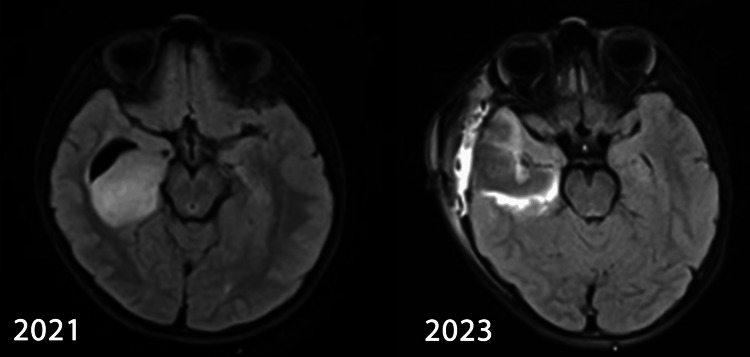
MRI of Patient 1 at baseline and most recent follow up
